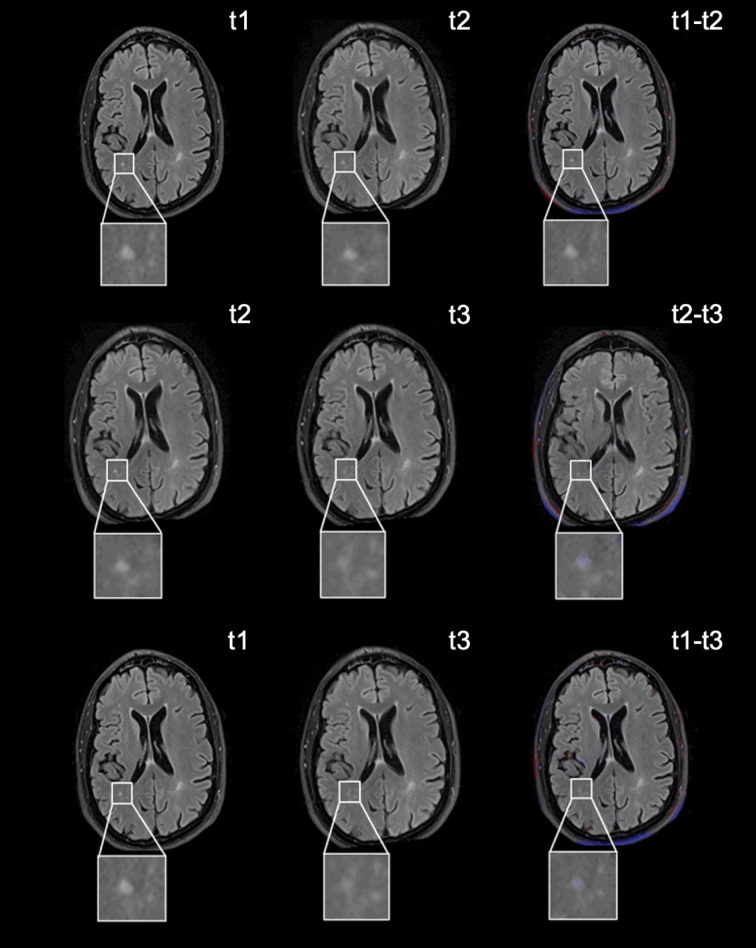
Semiquantitative analysis of white matter hyperintensity size between different time points. Analysis of fluid-attenuated inversion recovery (FLAIR) images using Longitudinal Brain Imaging (LoBI) application (IntelliSpace Portal, Philips Medical Systems, Best, The Netherlands). The blue color indicates a reduction in white matter lesion size, measured at 1 mm, located in the right parietal region, between timepoints 2 and 3 (t2-3) as well as between timepoints 1 and 3 (t1-t3).
